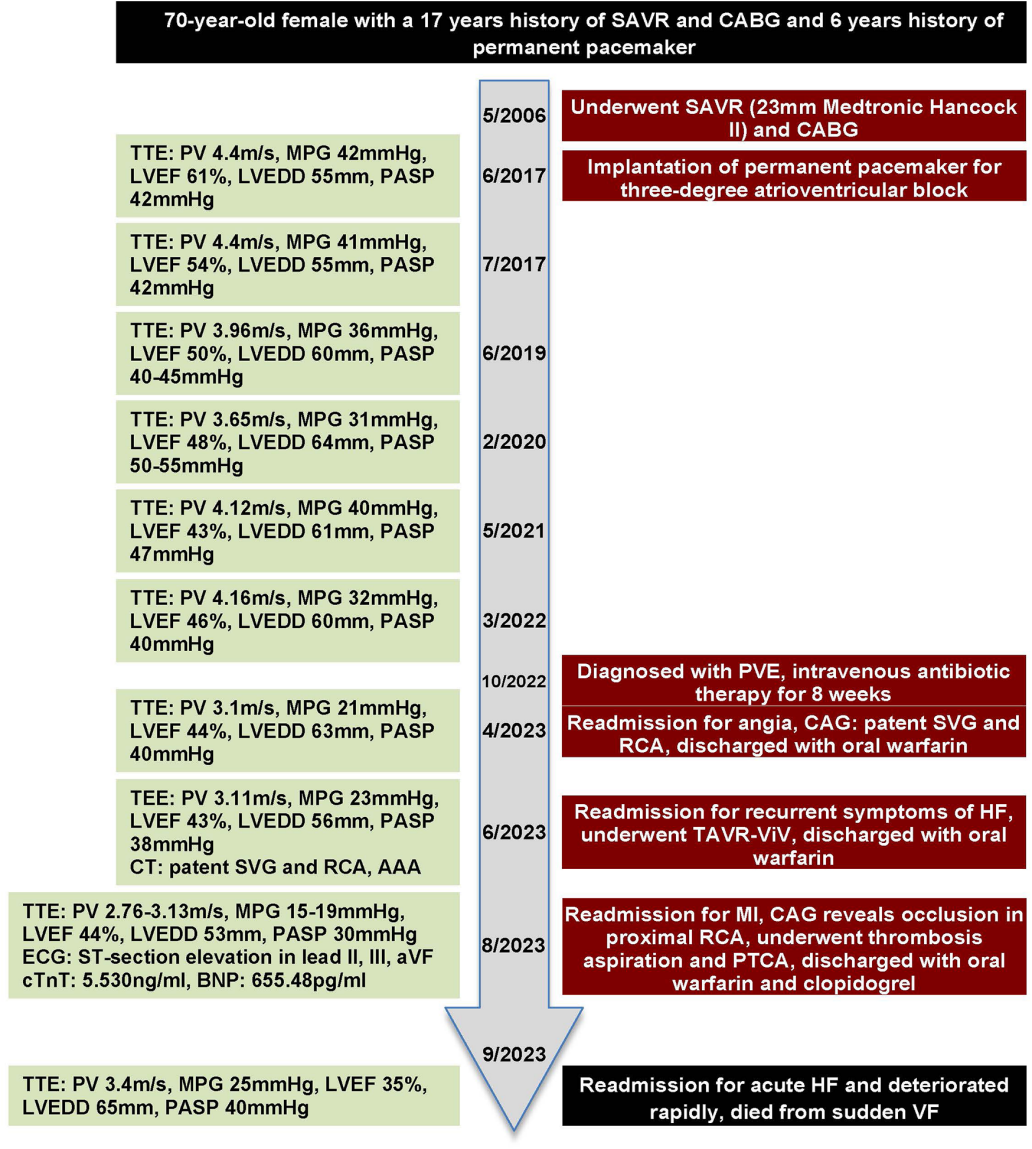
Timeline with key parameters of echocardiography and events throughout this case.
